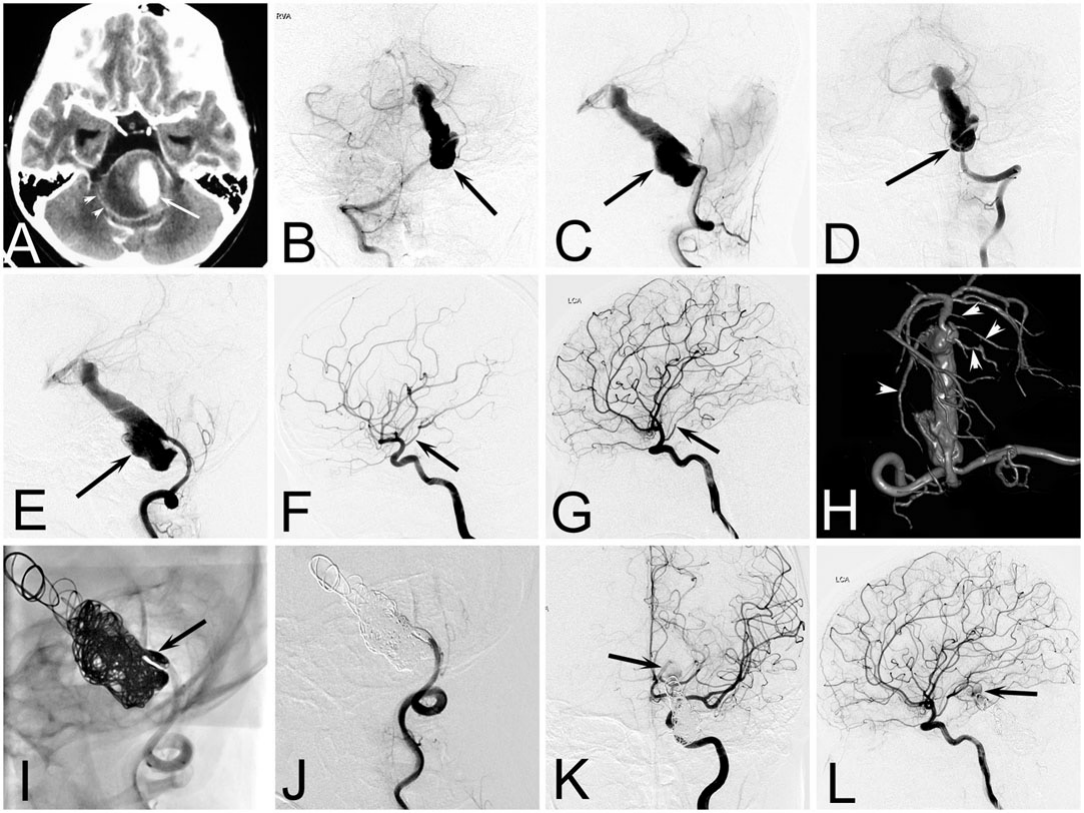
Case of a 12-year-old girl who presented with acute headache complicated with diplopia and neck rigidity. (A) Two-dimensional computed tomographic angiography showing a giant aneurysm of basilar artery with serious mass effect. (B–E) Right vertebral angiogram (B,C) and left vertebral angiogram (D,E) showing a dolichoectatic dissecting aneurysms of middle and lower segment of the basilar artery. (F,G) Right internal carotid artery (ICA) (F) and left ICA (G) angiogram showing the patency of bilateral posterior communicating artery (arrow). (H) A 3D reconstruction of a left vertebral angiogram showing the branches of upper segment of basilar artery (arrow heads). (I,J) The mask image (I) and immediate left vertebral angiogram (J) showing the lower part of the aneurysm as well as the distal end of left vertebral were completely embolized. (K,L) Immediate left ICA angiogram showing the left posterior cerebral arteries as well as upper segment of basilar artery (arrow) appearing *via* the left posterior communicating artery.
